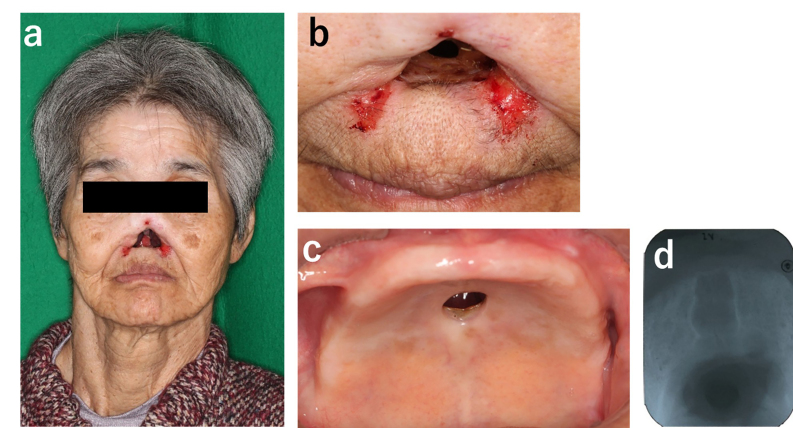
Clinical findings at the 9-year follow-up (2015 at age 76). (a) Facial photograph; (b) close-up view of the nasal defect; (c) maxillary intraoral photograph; (d) maxillary occlusal radiograph.
